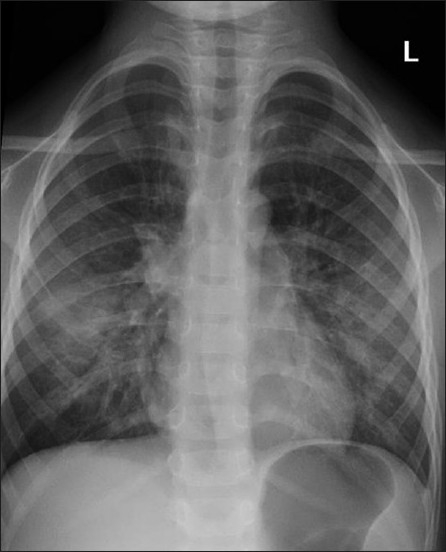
A chest X-ray of a patient with a CD4 count <200/mm^3^ showing perihilar ground-glass appearance in the shape of bats-wings
